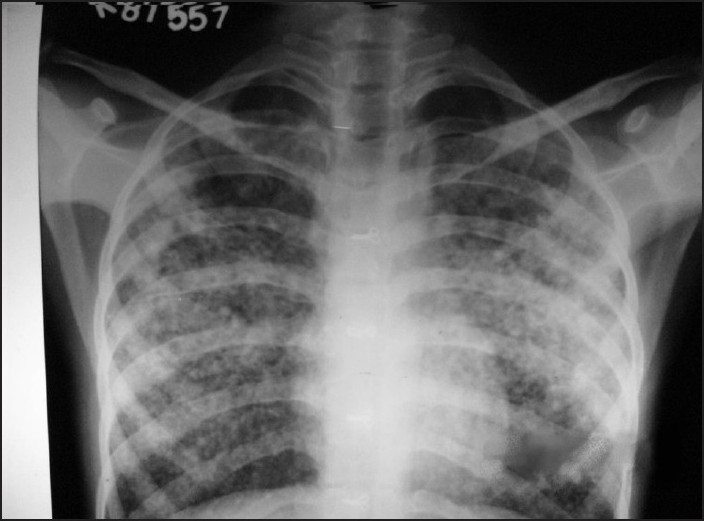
X-ray chest PA view showing pulmonary oedema
